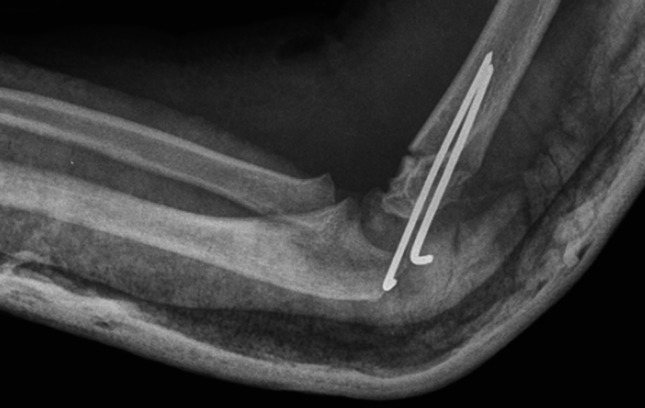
X-ray of third type of supracondylar humerus fracture after reduction—lateral view
